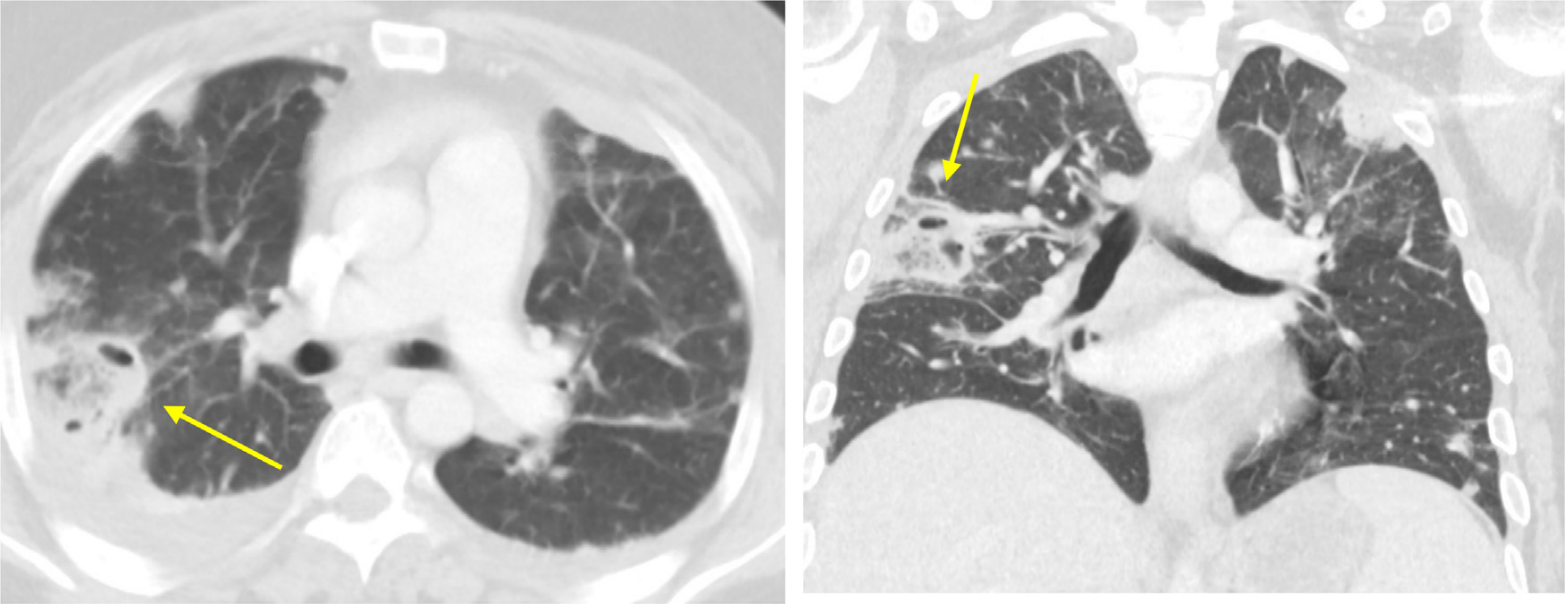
Axial (A) and coronal (B) images of CT scan show a large cavitary nodule in the right upper lobe, measuring 4.2 × 3.6 cm.
